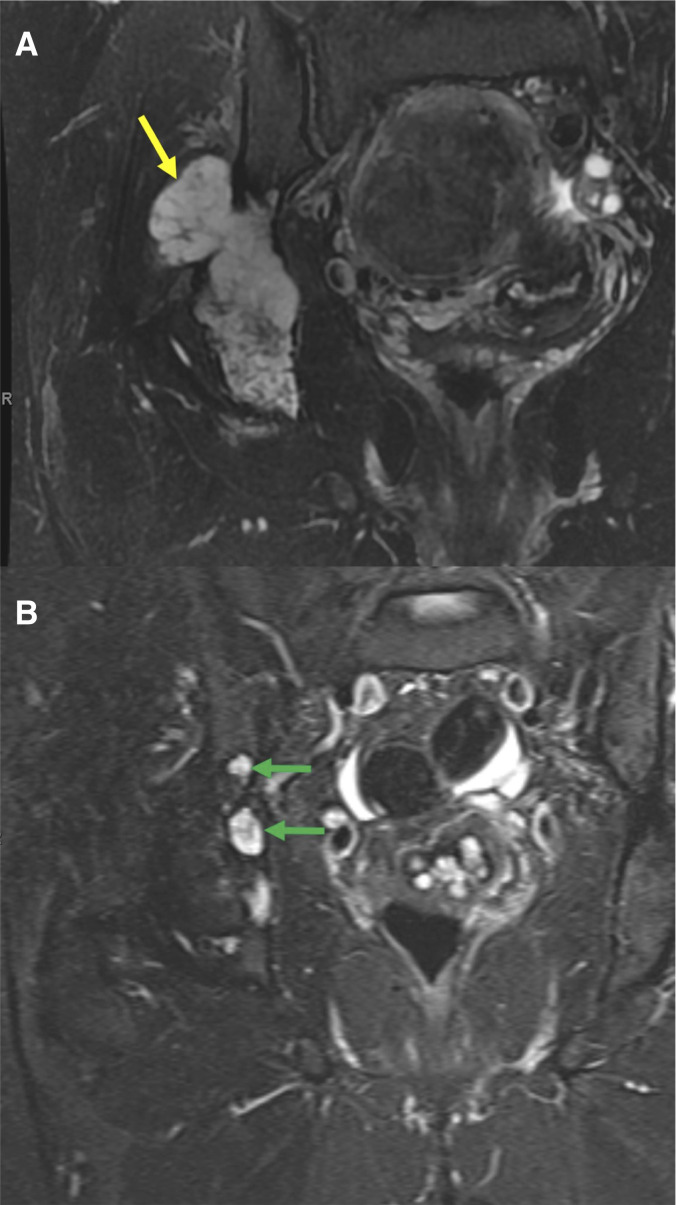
Radiographic images of a patient with conventional CS with PR to ivosidenib at baseline (A) and cycle 55 (B). Imaging of a 38-year-old female with grade 1 conventional CS (involving the right pelvis) previously treated with one systemic therapy, who then received ivosidenib. Coronal fat–suppressed T2-weighted MRI of the pelvis demonstrated a lobular CS in the right ilium and ischium, with relatively uniform hyperintensity, and a lateral extraosseous component at baseline (yellow arrow). After treatment with ivosidenib, there was reconstitution of the fatty marrow signal in the right ilium, with smaller residual islands of T2-hyperintense foci (green arrows) and regression of the extraosseous component. This resulted in a PR that lasted for 25.8 months.
